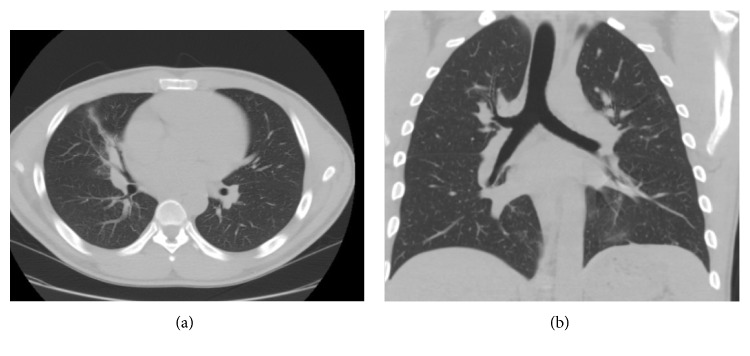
(a) CT chest (axial view) three months after initiation of treatment showing near-complete resolution of the right middle lobe infiltrate. (b) CT chest (coronal view) three months after initiation of treatment.
